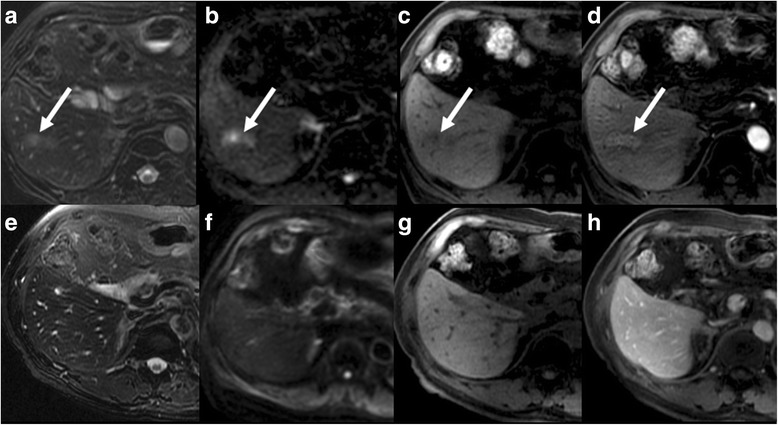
Case 4. MR images demonstrate an elongated lesion in the periphery of segment VI (*arrows*) with high SI on T2WI (a), restriction on DWI (b), low SI on T1WI (c), and post-contrast enhancement (d). The lesion resolved on follow-up MRI (e-h)
